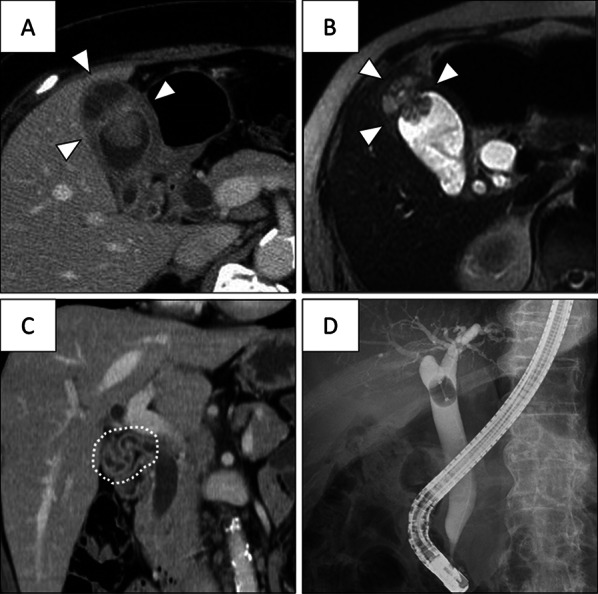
Enhanced computed tomography and T2-weighted magnetic resonance imaging. A, B A papillary or polypoid tumor was present in the gallbladder. C The gallbladder wall was generally thickened, and the thickening continued to the cystic duct. D Endoscopic retrograde cholangiography revealed a filling defect in the cystic duct
